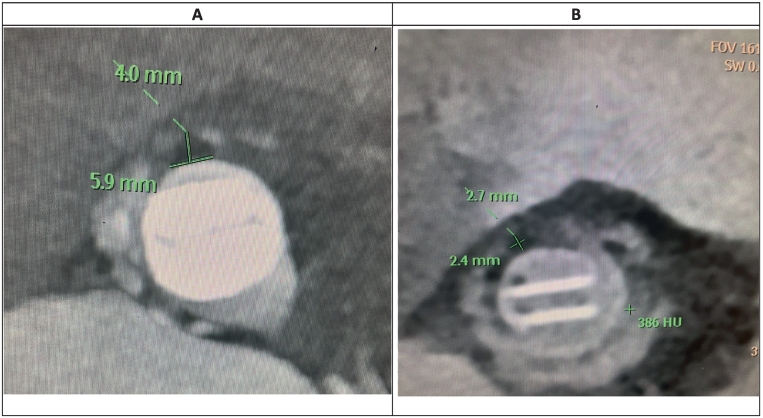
CT with contrast at baseline showed stuck right (posterior) disc with a thrombus (6 × 4 mm) surrounded by pannus formation (A) while repeat study after one cycle of thrombolytic therapy there was reduction in the thrombus size (2.7 × 2.4 mm) (B).
